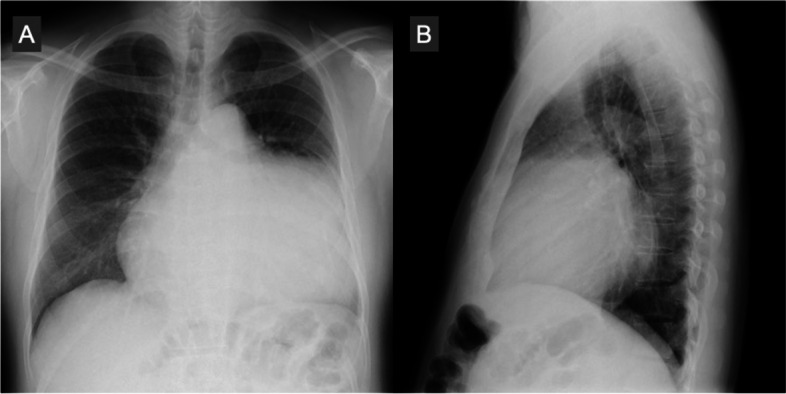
Preoperative chest X-ray. Chest X-ray shows cardiomegaly and a cardiac silhouette expands into the left thoracic cavity
